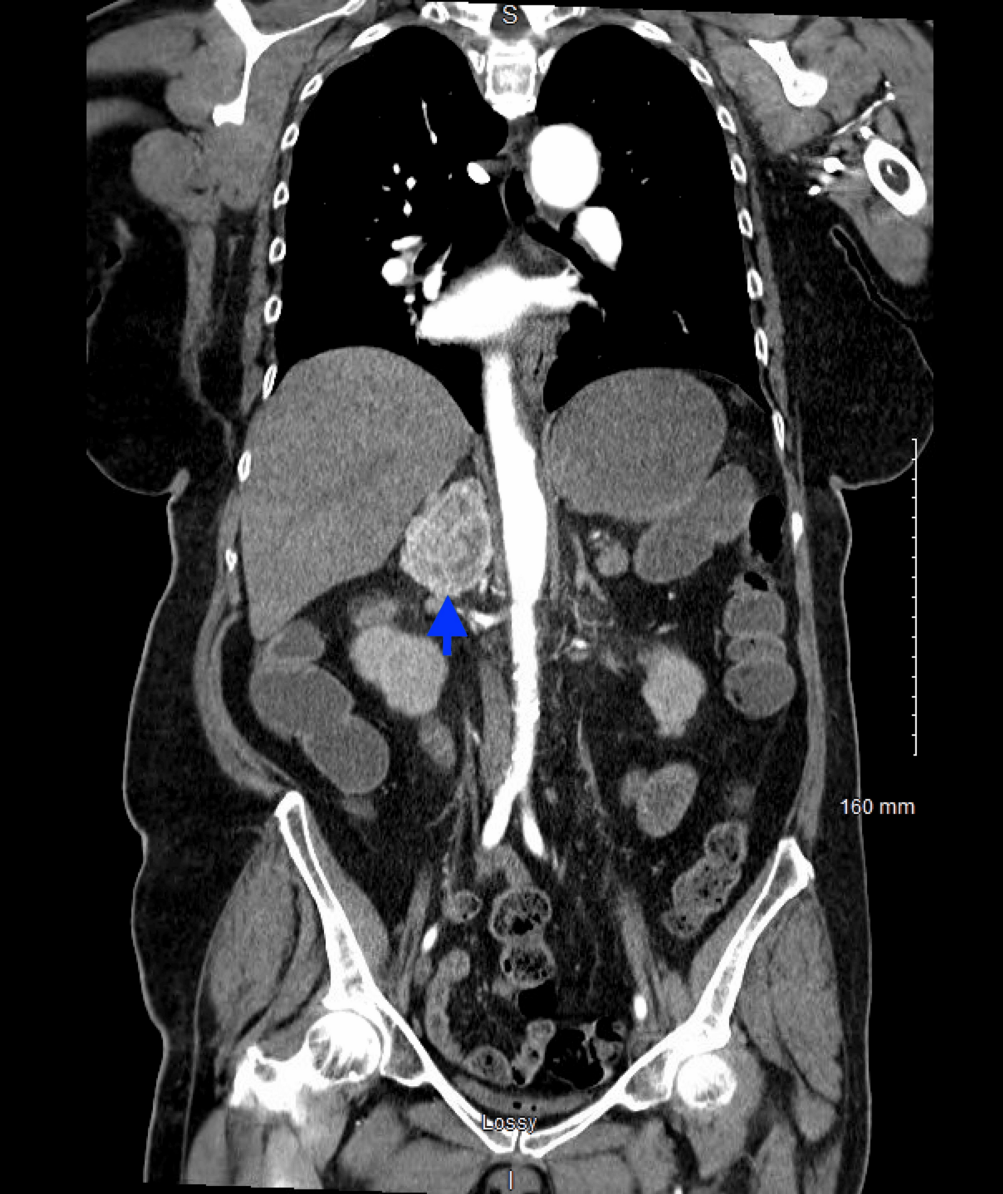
CT abdomen/pelvis with IV contrast (coronal view) CT shows an arterial enhancing mass lesion (blue arrow) in the right retroperitoneal space measuring 4.2 x 4.3 x 5.5 cm.
